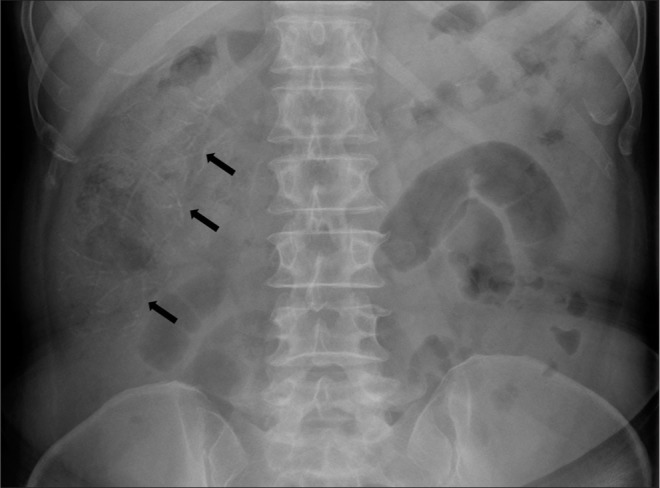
A 60-year-old female with fecal immunochemical test positive and recent onset (3–4 months) of intermittent abdominal pain with loose stools. Magnified abdominal radiograph showing faint threadlike linear calcifications (arrows) oriented along the ascending colon.
